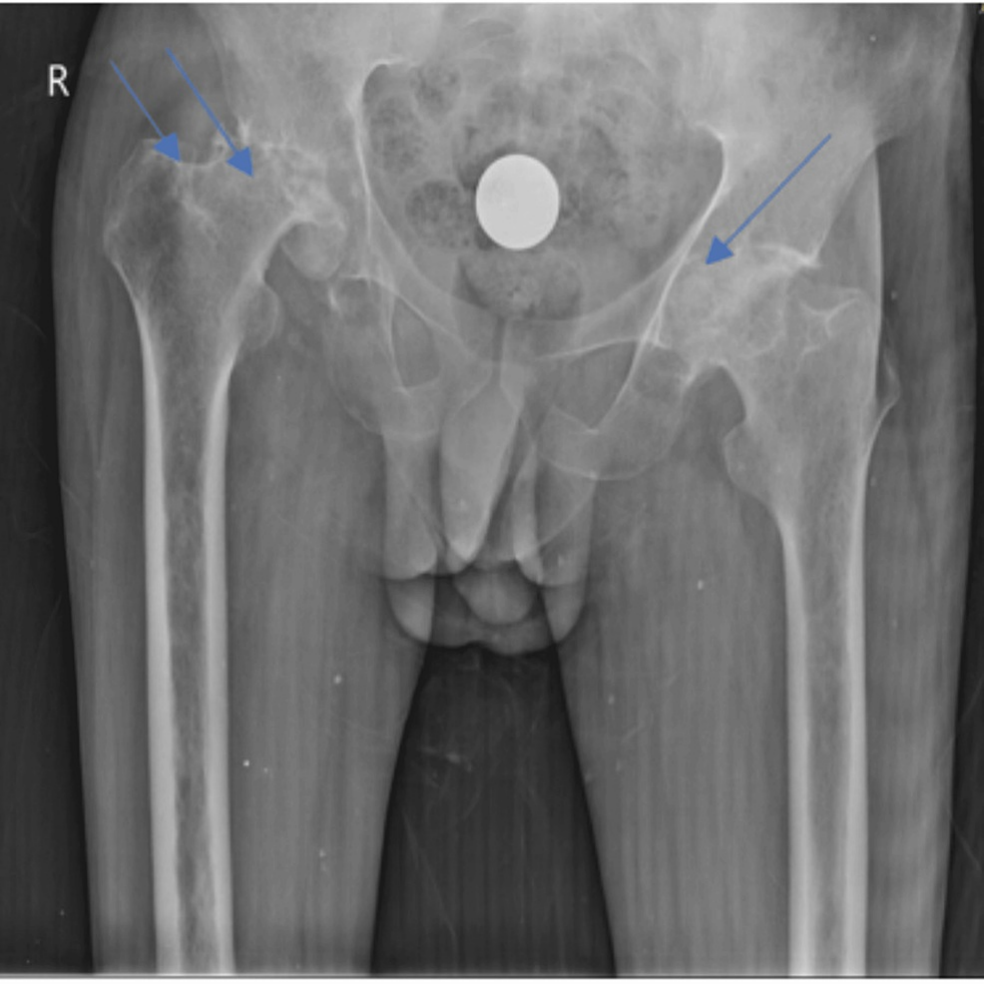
Preoperative X-ray of the pelvis and both hips showing (1) bilateral flattening and destruction with lytic changes of the femoral head; (2) loss of contour of the right and left femoral head; and (3) subchondral collapse and sclerosis of the bilateral femoral head.
